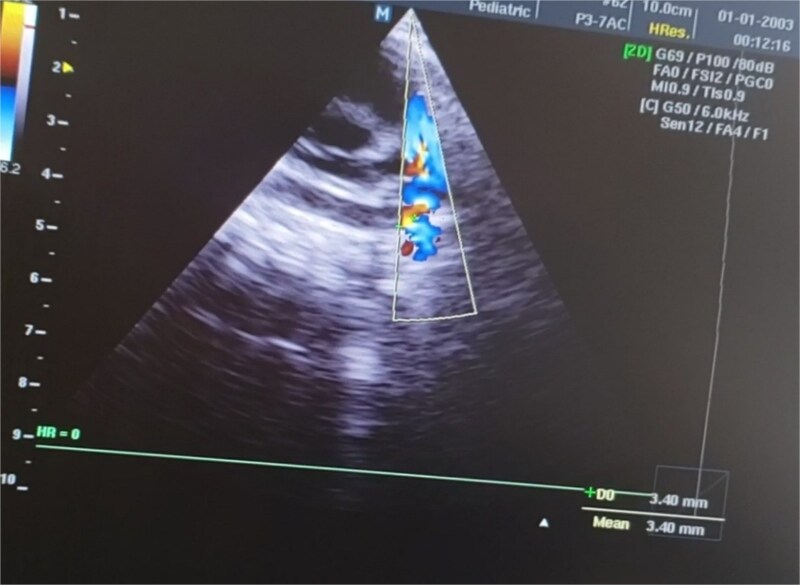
The Doppler echocardiography of the newborn baby shows blood flow from the aorta to the pulmonary artery, indicating a 3.4 mm patent ductus arteriosus. This anomaly contributed to the development of PPHN and hemodynamic instability of the infant.
